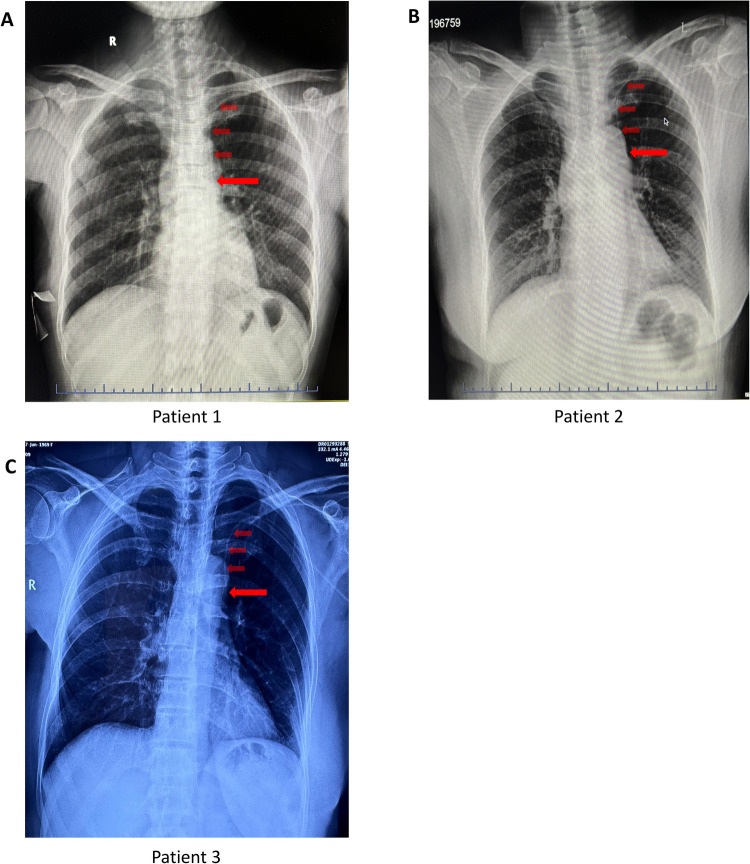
Postoperative chest X-ray of PLSVC patients.
